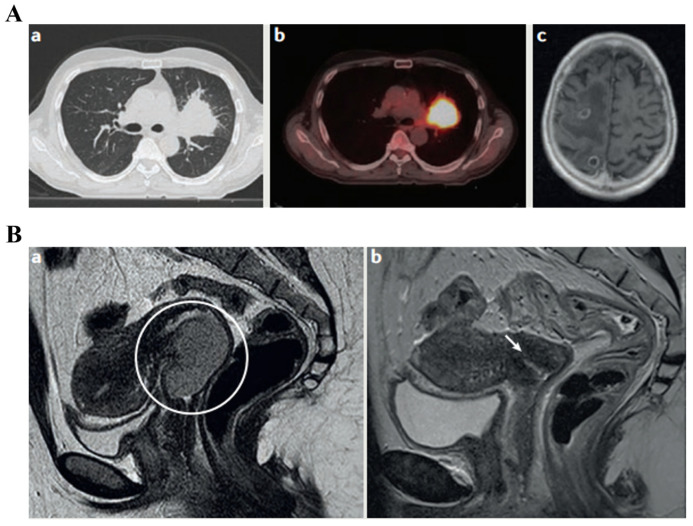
(A) The TNM staging of a patient diagnosed with stage IV non-small-cell lung cancer (T2 N0 M1) revealed the following: (a) a T2 tumor in the lung detected through CT imaging, (b) no signs of involvement in local lymph nodes based on PET-CT scans, and (c) the presence of brain metastases as observed in MRI scans. (B) (a) An individual with cervical cancer (T3b N0 M0) initially came with a sizable main tumor (shown inside the circle). (b) Nevertheless, following chemoradiation therapy, the patient showed a full recovery, and the cervix was returned to normal (the location of the remnant tumor is shown with the arrow) [51].
